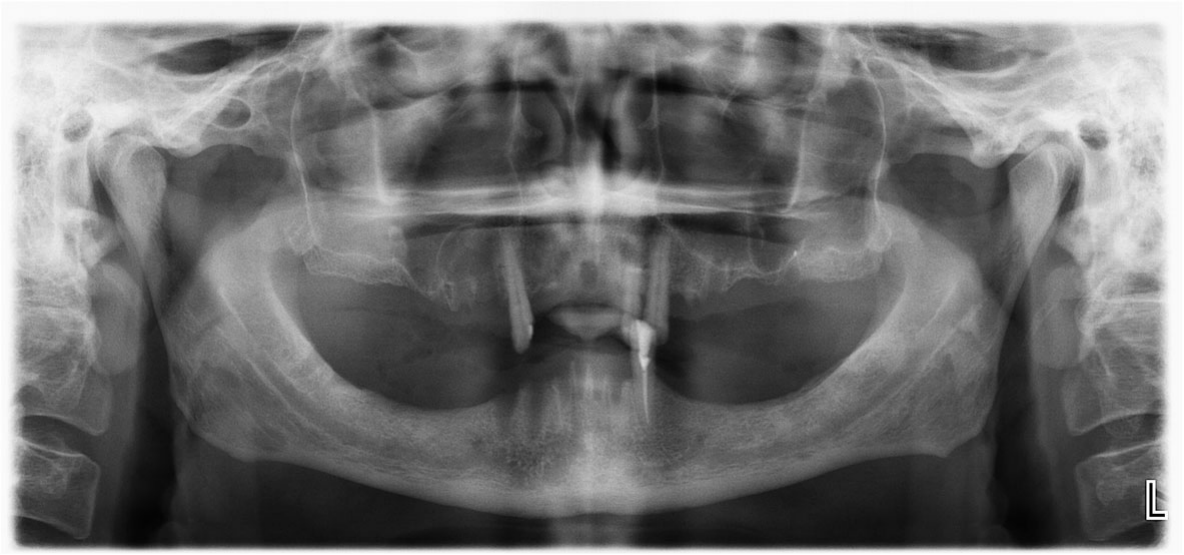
A panoramic radiography before surgery
